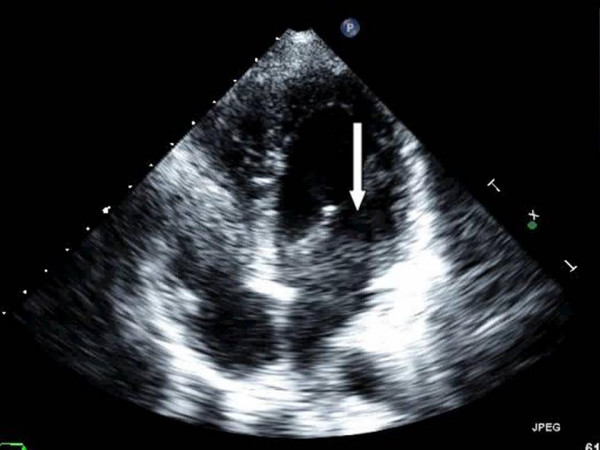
A large shadow is seen across the right and left ventricle caused by the silicone breast implant that limited the echocardiographic window in the 4 chamber view.
